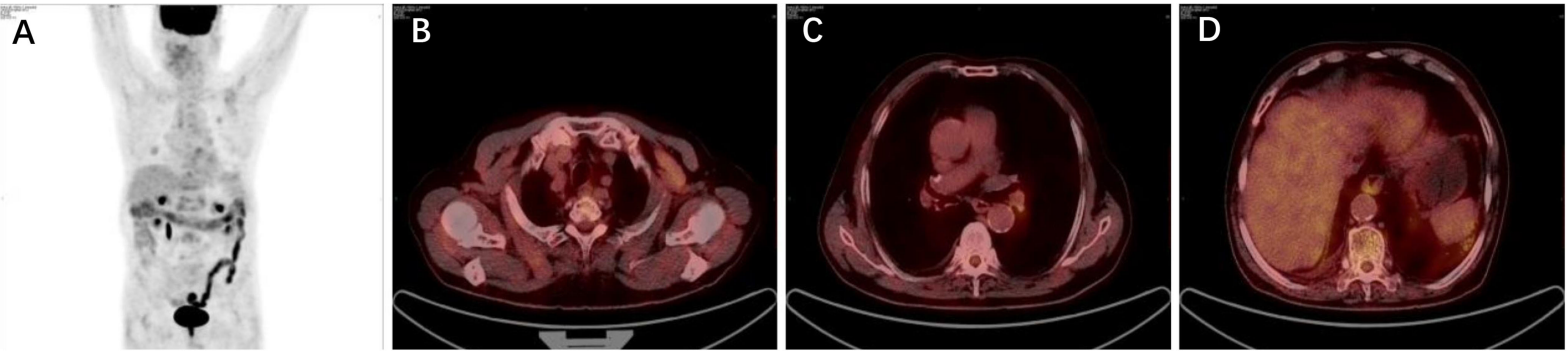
(A–D) Clinical and radiological CT of the last follow-up in October 2021.
